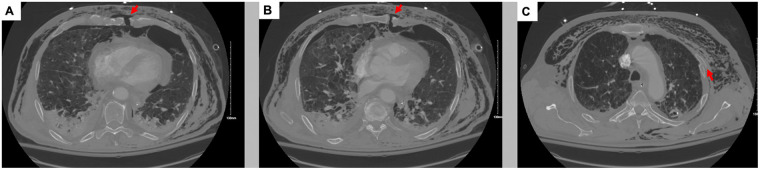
Concomitant rib and costal cartilage fractures. (A,B) Axial CT images show rib fractures with associated disruption of the anterior costal cartilage (red arrows). (C) Additional axial CT image demonstrates a rib fracture with adjacent subcutaneous emphysema (red arrow).
